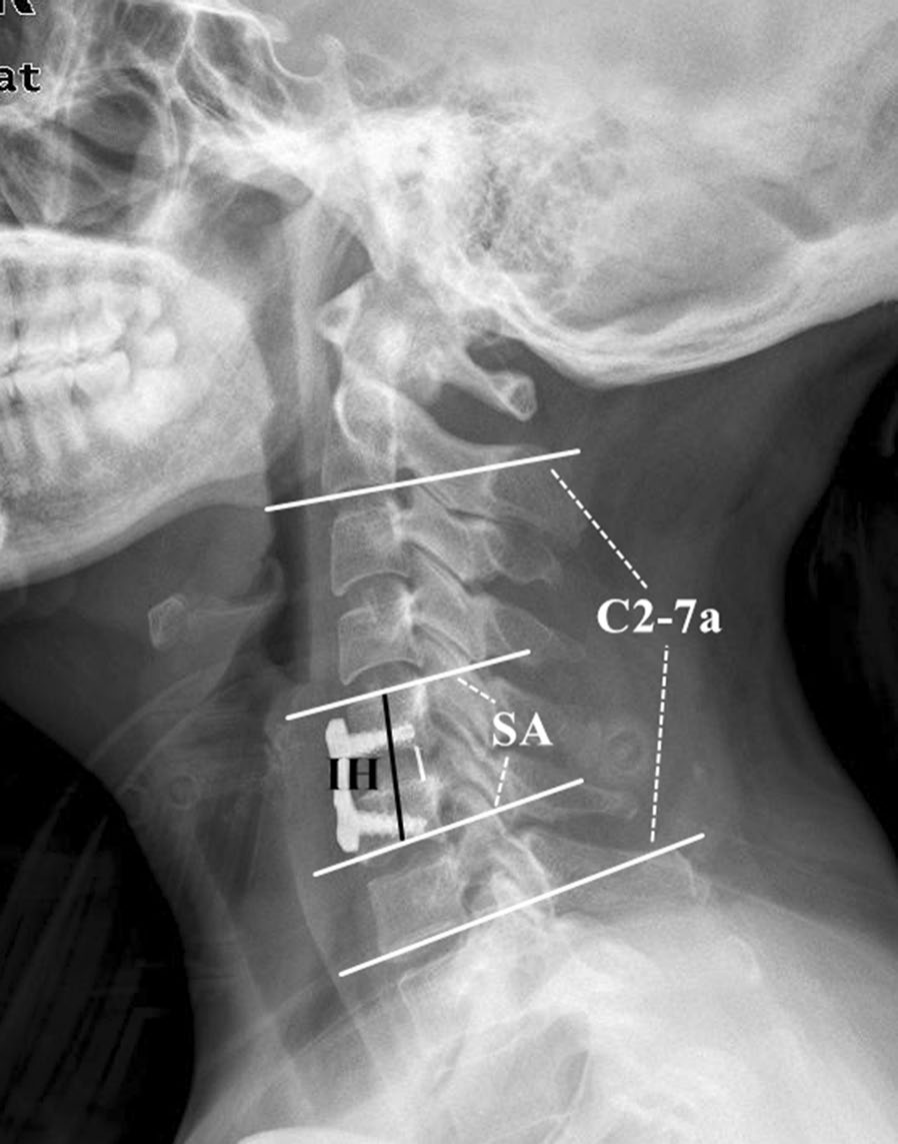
Representation of radiographic measurements: IH (intervertebral height), the distance from the midpoint of the upper endplate of the upper vertebral body to the midpoint of the lower endplate of the lower vertebral body; C2-7a (C2-7 angle), the Cobb angle between the lower endplate of C2 and C7; SA (segmental alignment), the Cobb angle between the superior endplate of the upper vertebra and the inferior endplate of the lower vertebra of the implanted level
